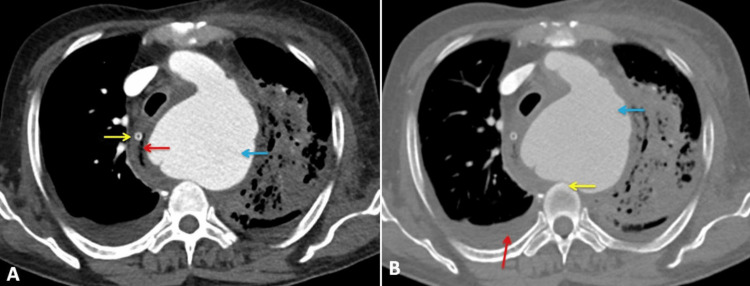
Computed tomography of the thorax. A (axial view): Aortic aneurysm (blue arrow), right side shift of the esophagus containing Ryle’s tube (yellow arrow), and probable site of fistula formation between aneurysm and esophagus. B (axial view, bone window): Aortic aneurysm (blue arrow), contact of the aneurysm with the vertebral body (yellow arrow), and pleural effusion (red arrow)
